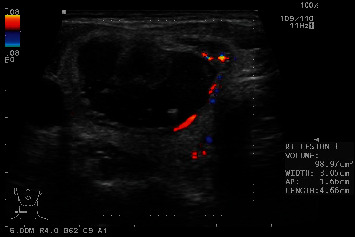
Ultrasound image before presentation showing the right mid to lower pole mixed cystic-solid thyroid nodule measuring 4.66 × 3.05 × 1.66 cm, with a well-defined margin and comet-tail artefact (consistent with the colloid nodule).
